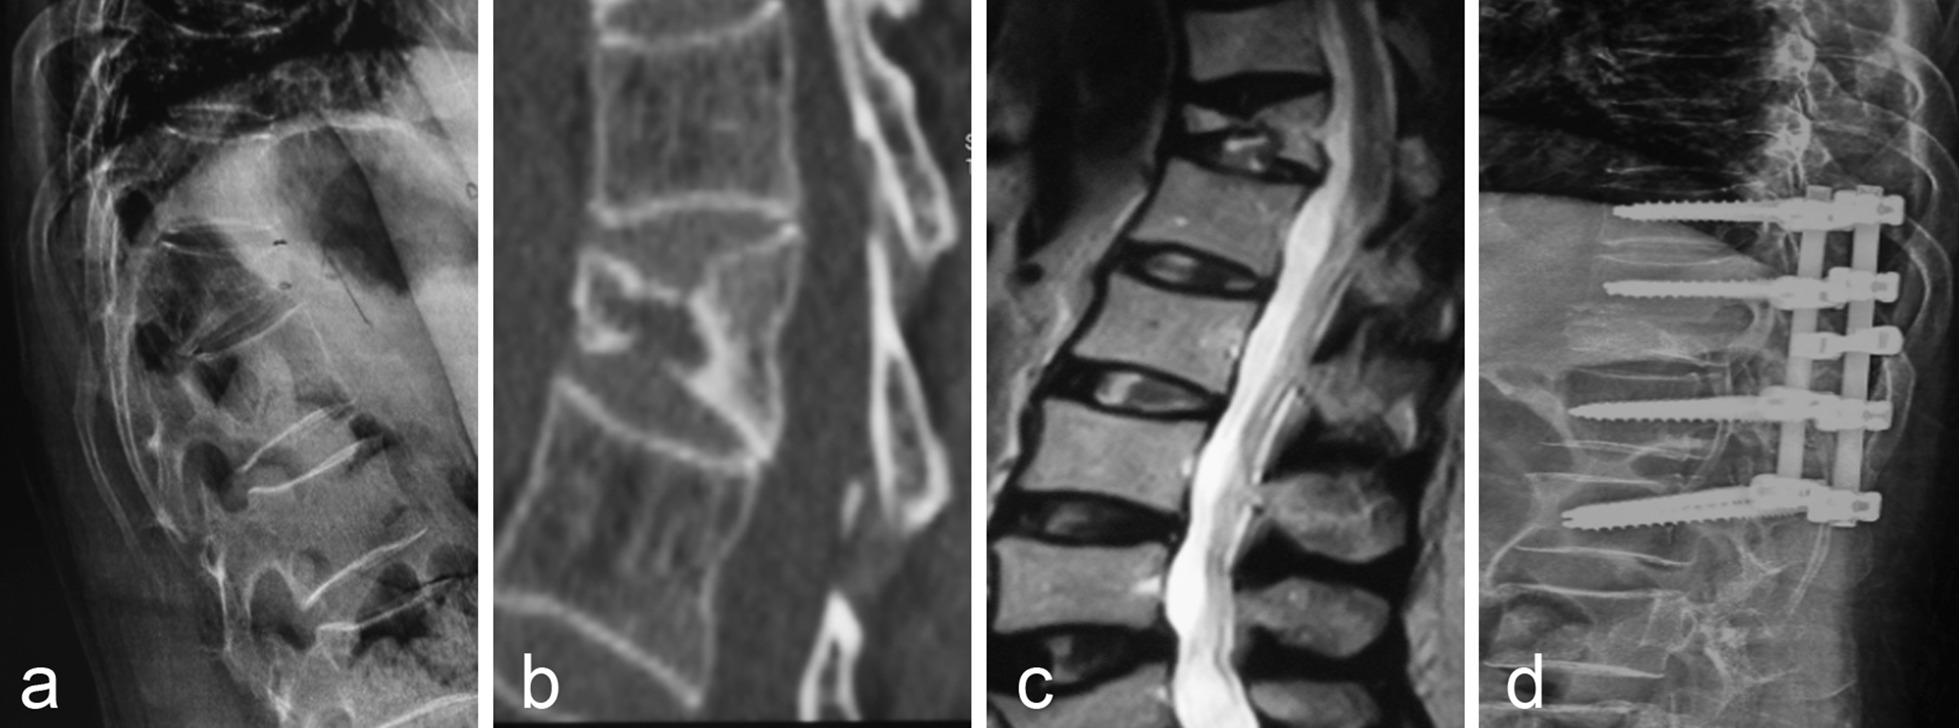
A 63-year-old female patient (Grade IV) presented with minor trauma 10 months ago and low back pain 2 months ago, which was associated with activity. a Thoracic X-ray showed a height loss of the T12 vertebral body and kyphosis of the thoracolumbar segment; c MRI T2 image showed low signal in the T12 vertebra; d Postoperative X-ray showed that the patient underwent posterior osteotomy and fusion internal fixation
